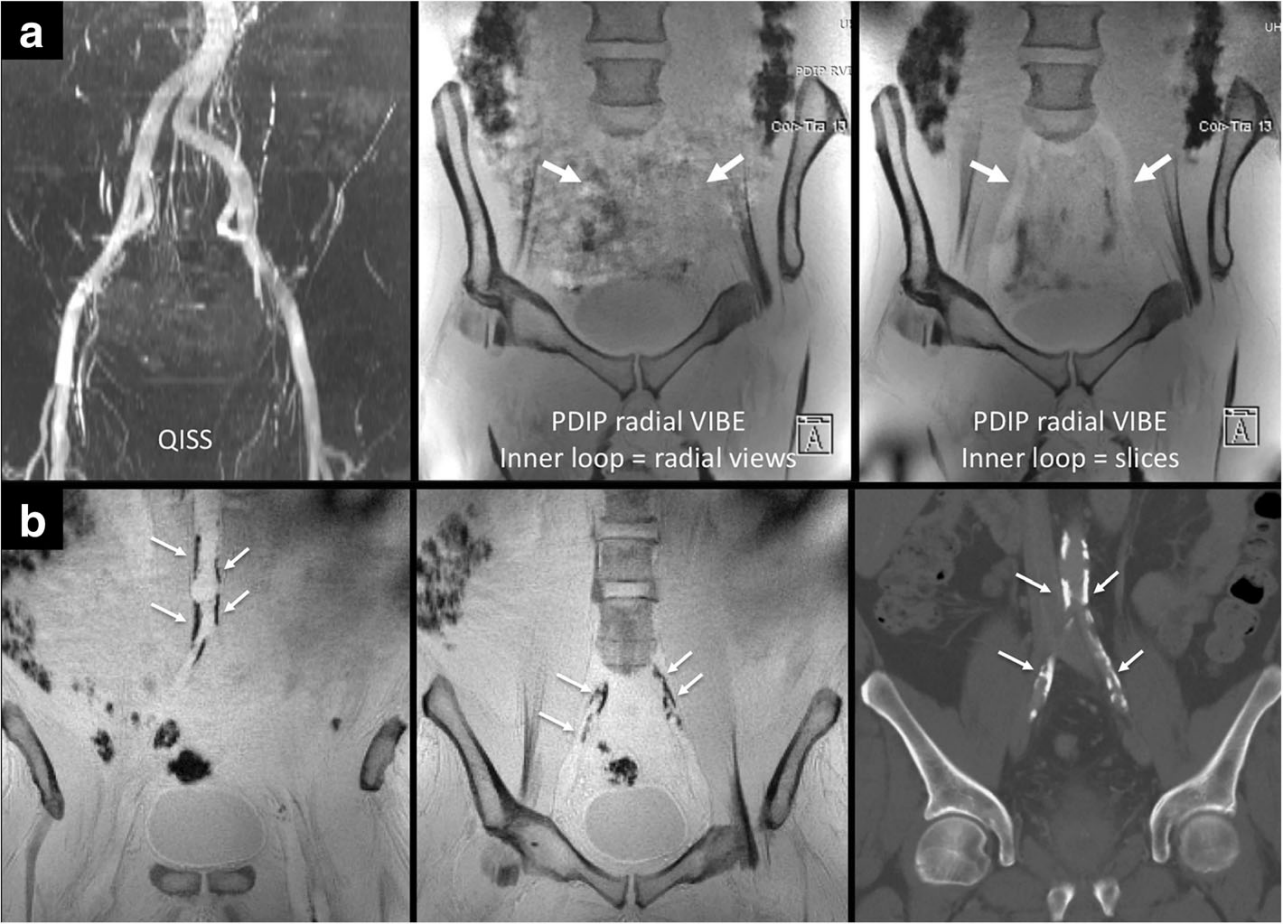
Evaluation of the aorto-iliac vessels using PDIP-SOS CMR. (a) Healthy volunteer. Left: QISS nonenhanced CMR angiogram used for positioning the stack of stars imaging slab. Middle: PDIP-SOS CMR using the legacy k-space encoding approach of acquiring all radial views in rapid sequence. There are severe ghost artifacts arising from centrally-located bowel loops that obscure the iliac vessels (arrows). Right: Identical PDIP-SOS acquisition except that all slice partitions (instead of radial views) were acquired in rapid sequence. The bowel-related artifacts are eliminated, so that the iliac vessels are well shown (arrows). Note that both the aorto-iliac and ilio-femoral regions are encompassed in the field of view, allowing simultaneous assessment of both regions. (b) Patient with aorto-iliac calcifications. Left, middle: 4-mm thick minimum intensity projections from PDIP-SOS CMR clearly depict vascular calcifications involving the distal aorta and proximal iliac vessels. Right: Thin-slab maximum intensity coronal projection from the CT shows excellent correspondence with the PDIP-SOS CMR
